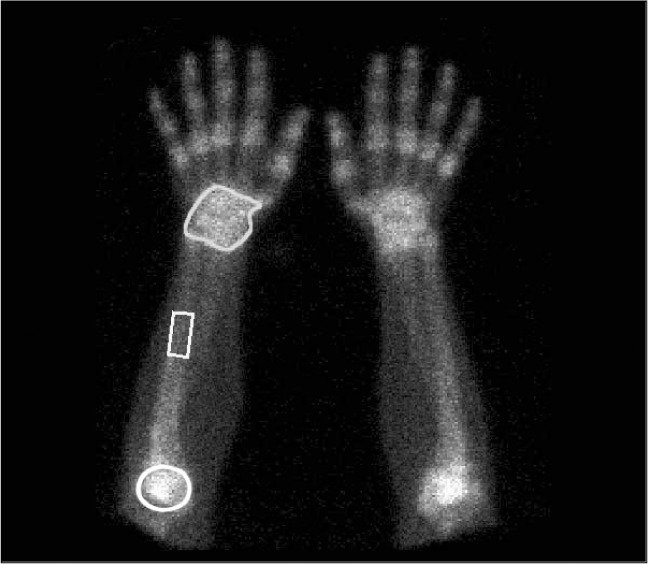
For evaluation of the elbows and wrists, regions of interest (ROIs) were outlined around the joints, bilaterally, and the ulnar shaft was used as the reference in the analysis with three-phase bone scintigraphy.
